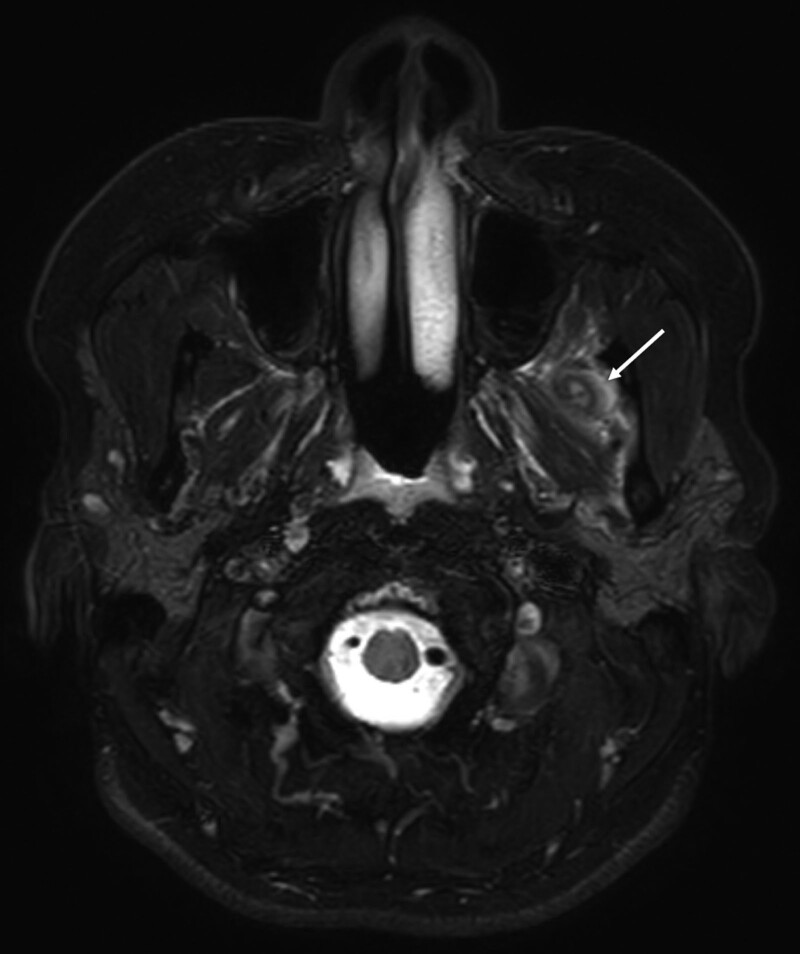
Fat-suppressed axial T2-weighted MR image shows a heterogenous hyperintense T2 signal lesion in the left temporal muscle near the medial aspect of the left mandibular ramus and the coronoid process, indicating myositis of the left temporal muscle. MR = magnetic resonance.
